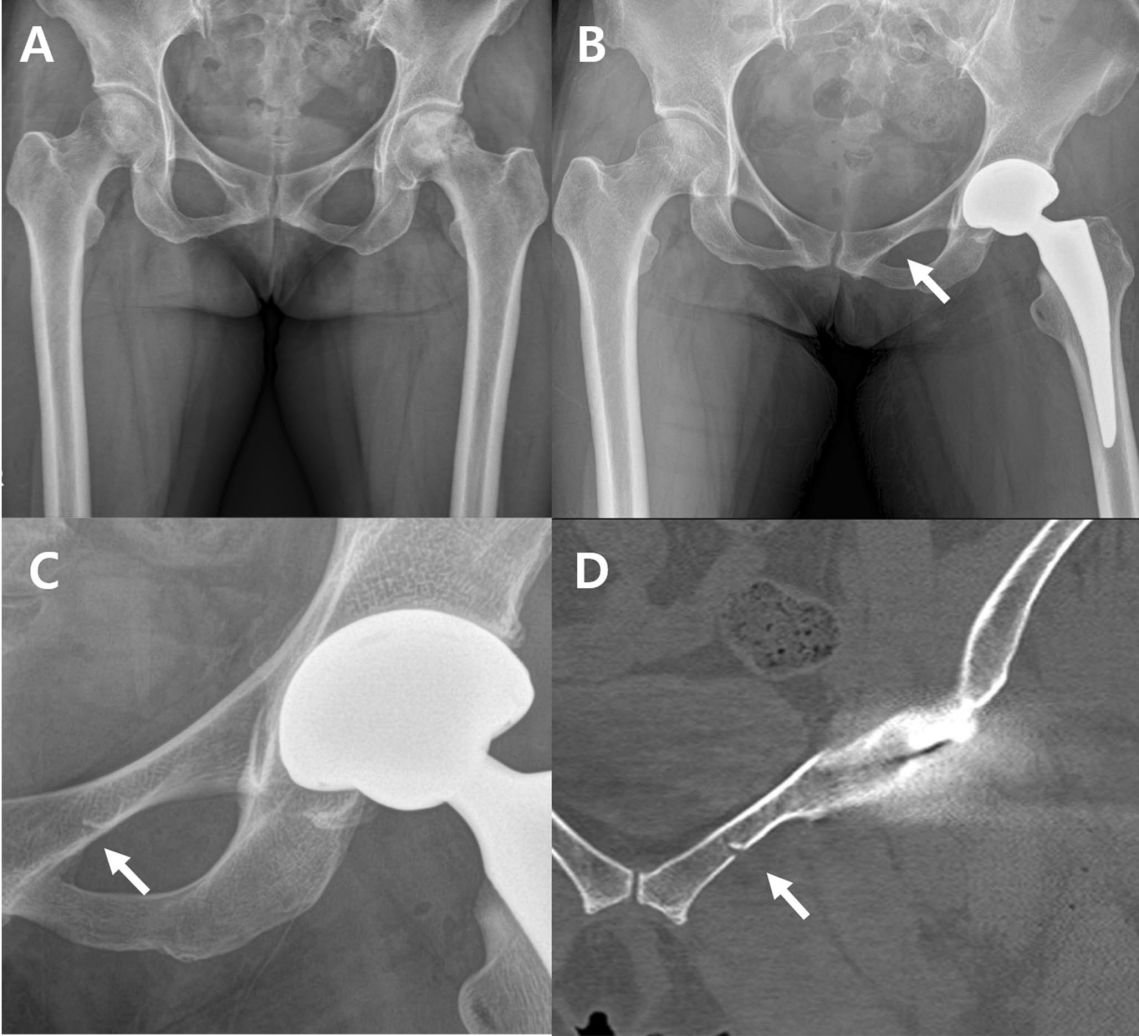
Radiographs showing superior pubic ramus fracture during total hip arthroplasty. (A) Preoperative anteroposterior view. (B) incomplete fracture lines on the left superior pubic ramus are visible on the postoperative hip anteroposterior radiograph. (C) Magnified view. (D) The postoperative hip computed tomography coronal view shows an incomplete fracture line on the left superior pubic ramus
